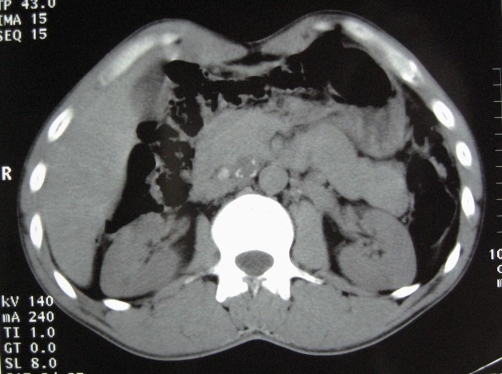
Computed tomography; retroperitoneal cystic mass, spontaneously hyperdense, containing calcifications.
